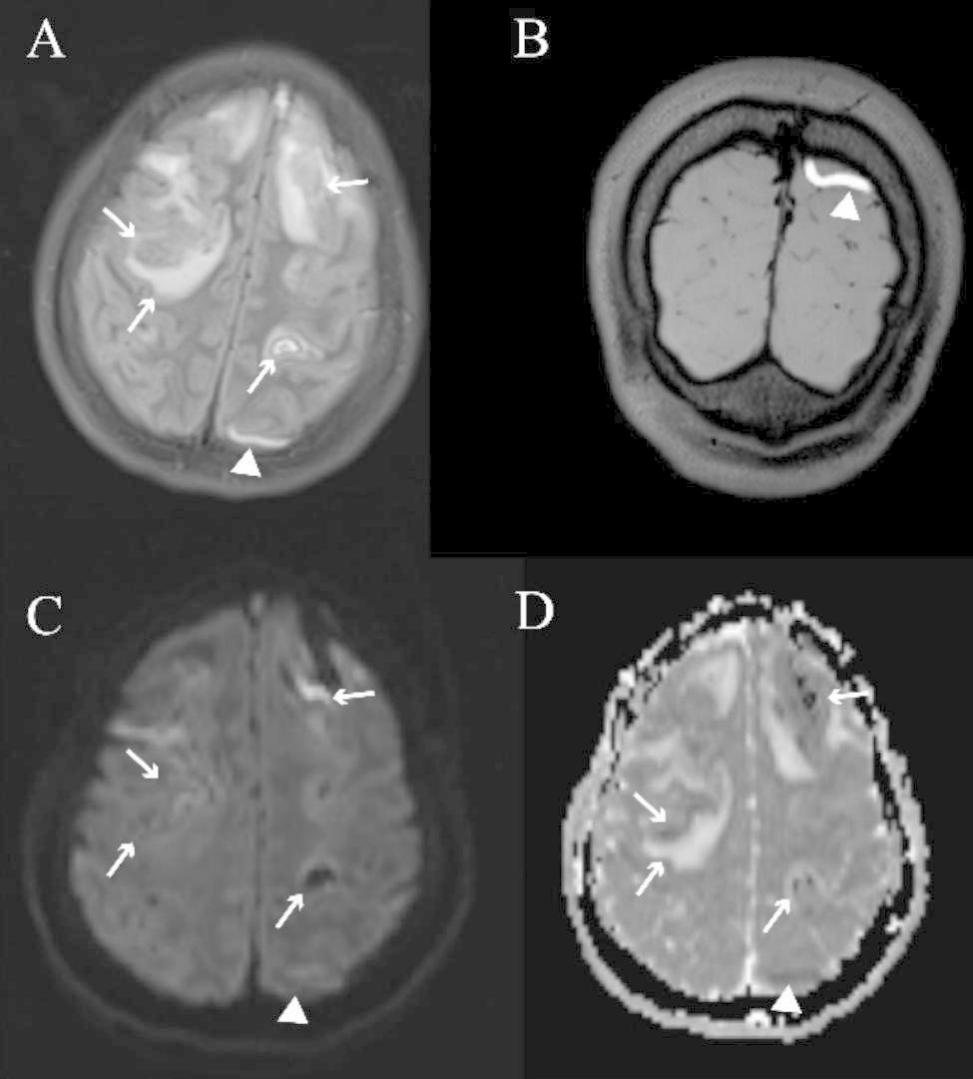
Typical images of cortical vein thrombosis on conventional magnetic resonance imaging and 3D-T1-SPACE scans in a 17-year-old female patient with cerebral venous sinus thrombosis. The fluid-attenuated inversion recovery (FLAIR, A) image shows a cord-like hyperintensity (white arrowheads, representing a subacute thrombosis) of the left cortical vein, which presents as restricted diffusion (white arrowheads) on the diffusion-weighted image (DWI, C) and apparent diffusion coefficient map (ADC, D), consistent with the thrombosed cortical veins on 3D-T1-SPACE (white arrowheads, B). Venous infarction and parenchymal hemorrhage can be observed as mixed signals on FLAIR, DWI, and ADC scans (white arrow)
